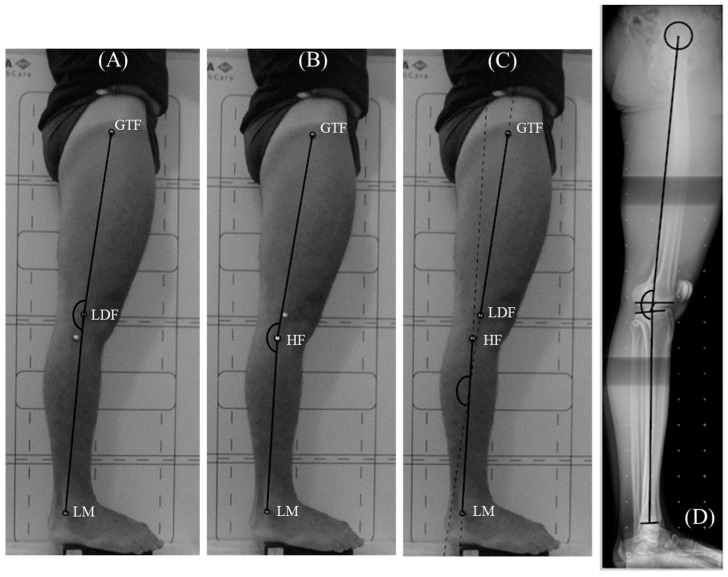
Sagittal knee angles calculated via photogrammetry: (A) condylar angle (CA); (B) fibula head angle (FHA); (C) four-point angle (4PA); and X-rays (D).
